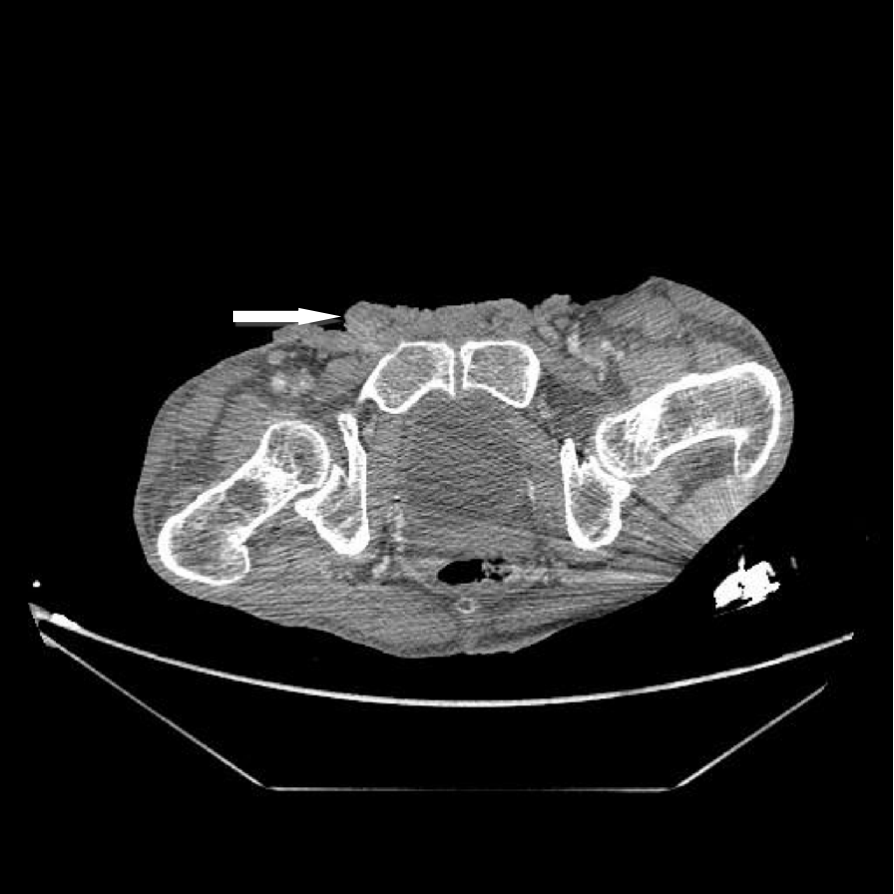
Computed tomography of the abdominal-pelvic floor showing the tumor invading the suprapubic region (white arrow).
